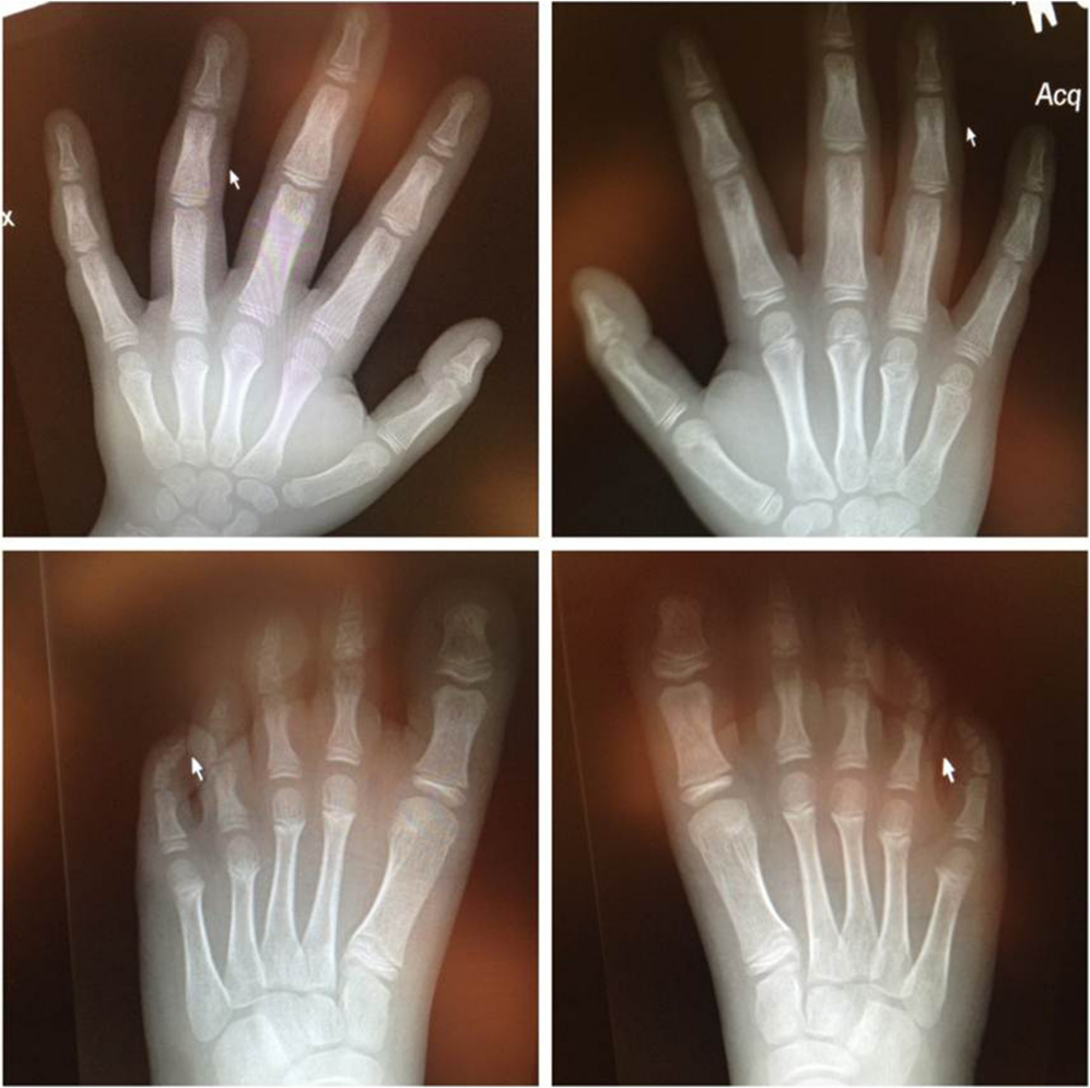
Radiographs showing digit anomalies of the proband. Anomalies included brachydactyly, wide nail beds, deviation of fourth digits to the ulnar side, radial displacement of thumbs, and clinodactyly of the fourth and fifth digit tarsal.
